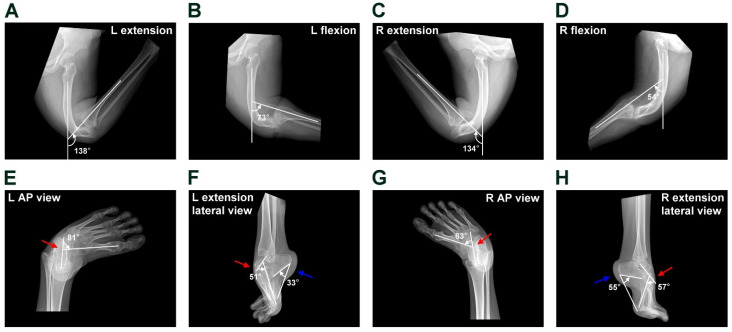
Pre-operative radiograph of the knees and feet. Both knee dynamic x-rays show the recurvatum deformity with various angles in extension and flexion (A–D). The foot and ankle anteroposterior (AP) and extended lateral view show talipes equinocavovarus with various angles of AP Meary’s angle (red arrow), lateral Meary’s angle (red arrow) and Calcaneal pitch (blue arrow) (E–H). The angles are indicated in the images.
